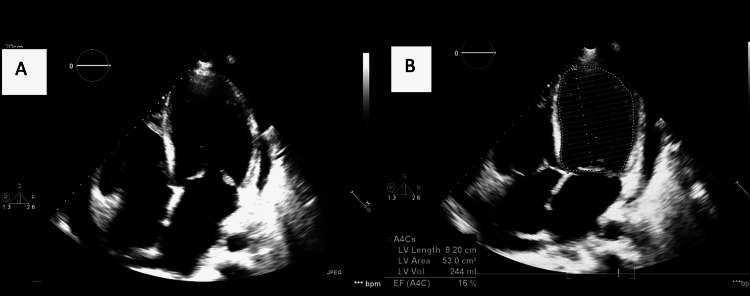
A. Transthoracic echocardiography (apical four-chamber view) shows severe LV dilation and dysfunction; B. Estimated EF using Simpson’s method LV: left ventricle, EF: ejection fraction
